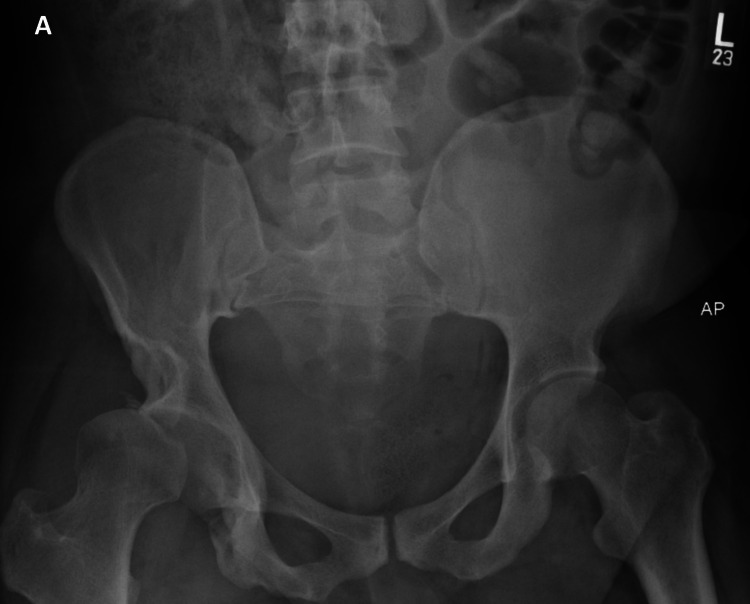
AP X-ray of the pelvis. This image shows superior subluxation of the right femoral head in the acetabulofemoral joint. Noted is the shallow nature of the right acetabulum compared to the contralateral side.
